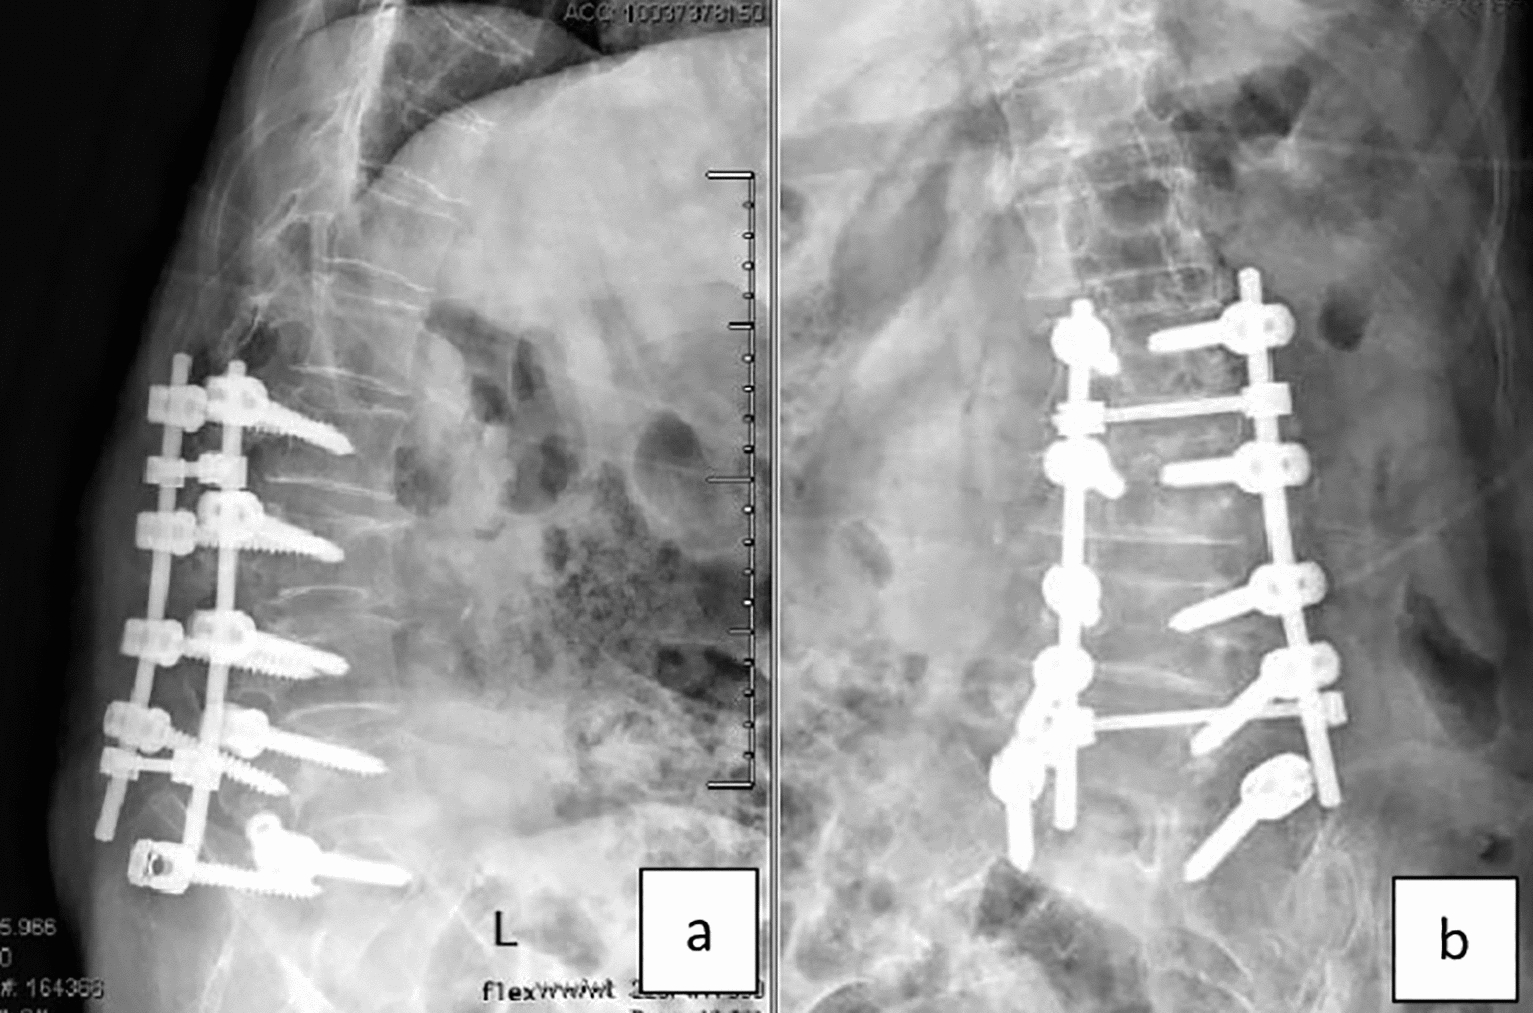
a, b Lateral X-ray shows screw head opening
